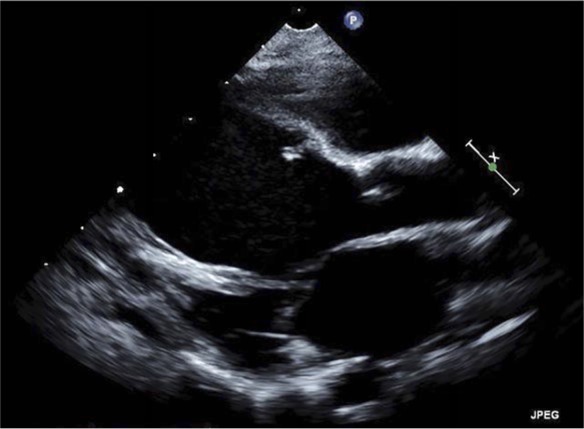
Two-year follow-up transthoracic echocardiogram (parasternal long axis view at end-diastole) demonstrated myocardial thinning with left ventricular (LV) dilation but overall decrease in echogenicity; LV systolic function was moderately depressed.
